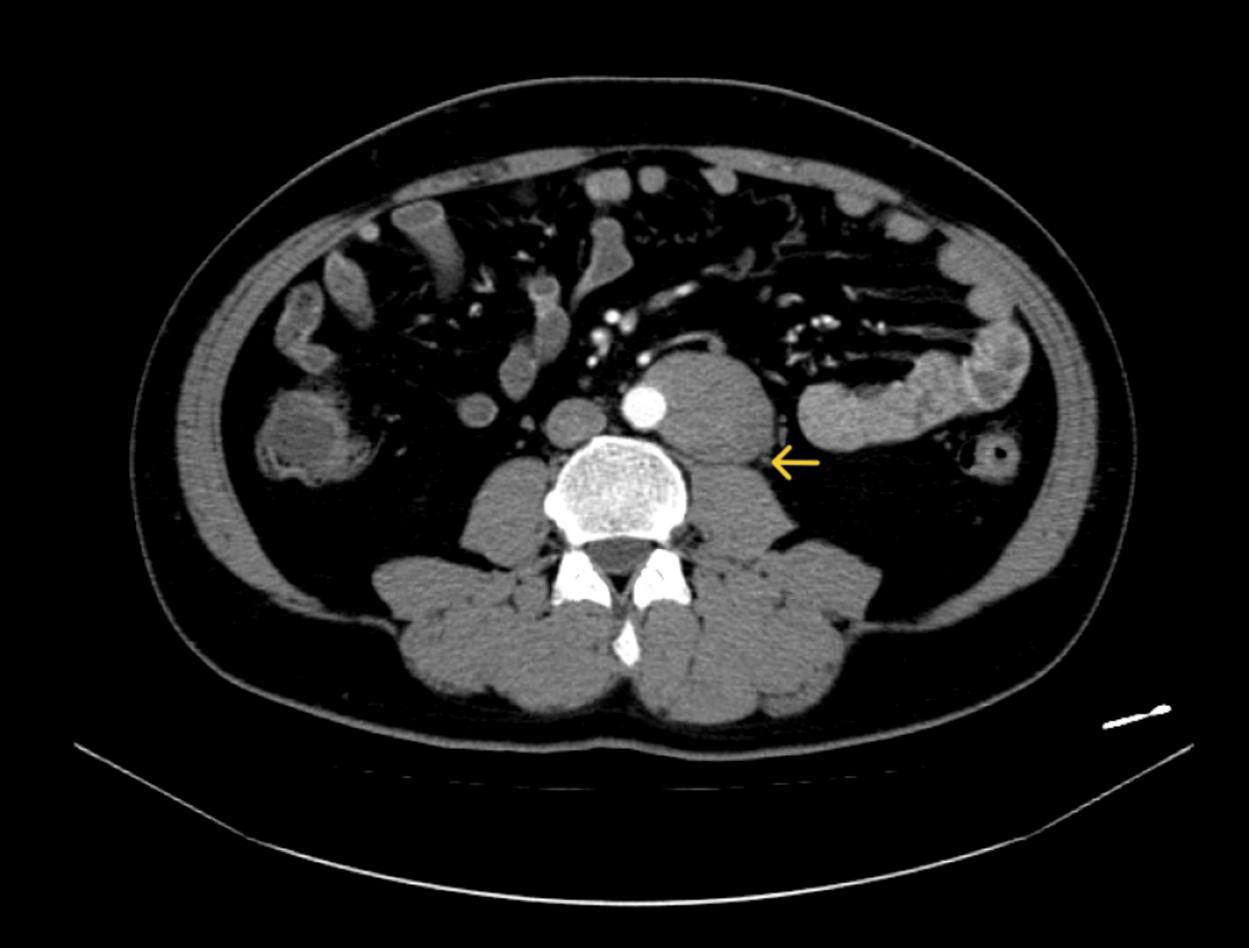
Preoperative contrast CT scan A 65 mm-long mass semi-peripheral to the aorta is seen on the left side of the aorta. There is no intervening fat layer between the aortic wall and the tumor. The tumor is in contact with the left ureter (arrow) CT: computed tomography
